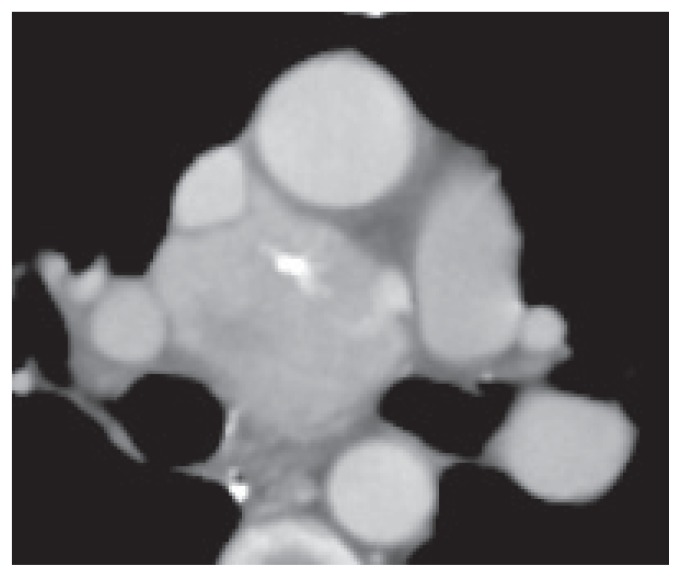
Post contrast axial CT image of the Castleman mass. Enhancement is fairly uniform and again a calcified focus is seen. Even though this is an arterial phase acquisition the intense vascularity of the mass is less well appreciated than on the dynamic MRI sequence (Fig. 2 images/movie). Note that this image was acquired 8 years after that shown in Figure 4, demonstrating the lack of disease progression in this patient.
